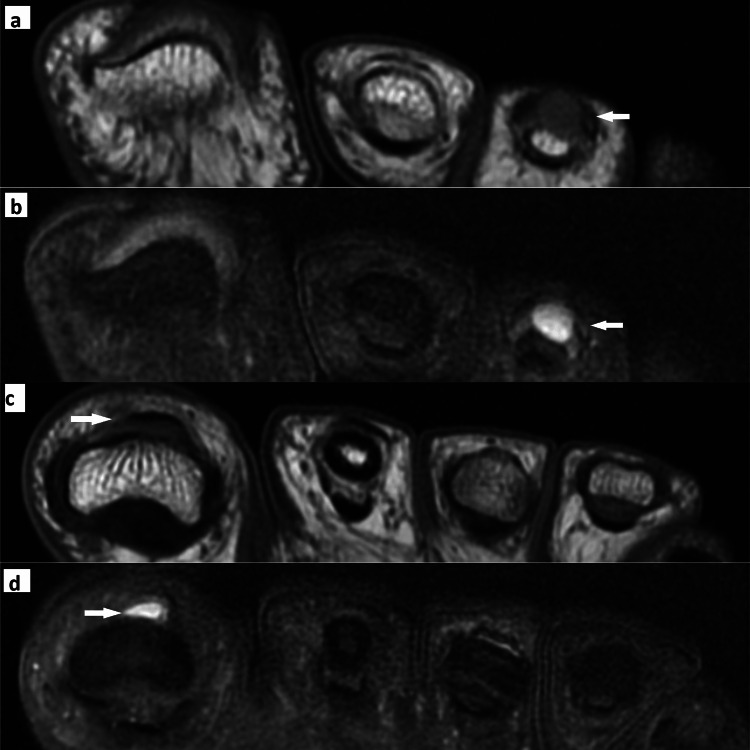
MRI features of the third toe swelling Axial T1-weighted image (a) shows an isointense lesion in the subungual aspect of the third toe and it appears hyperintense on the axial T2 fat-saturated (FS) image (b). The same patient's axial T1-weighted image (c) shows an isointense lesion in the subungual aspect of the great toe, and it appears hyperintense on the axial T2 FS image (d)
